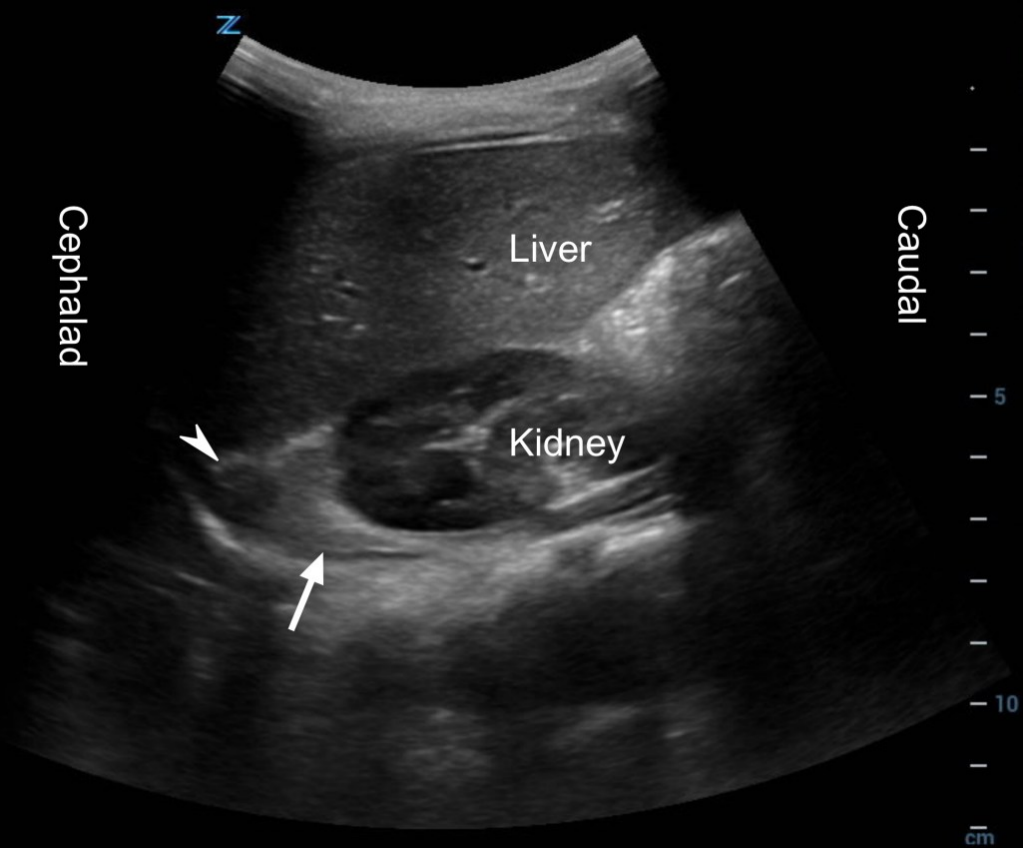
A focused assessment with sonography in trauma (FAST) right upper quadrant (RUQ) standard view. A prominent adrenal gland area (arrow) and an oval finding (arrowhead), later characterized as a blood clot, are seen in the cephalad pole of the kidney.
